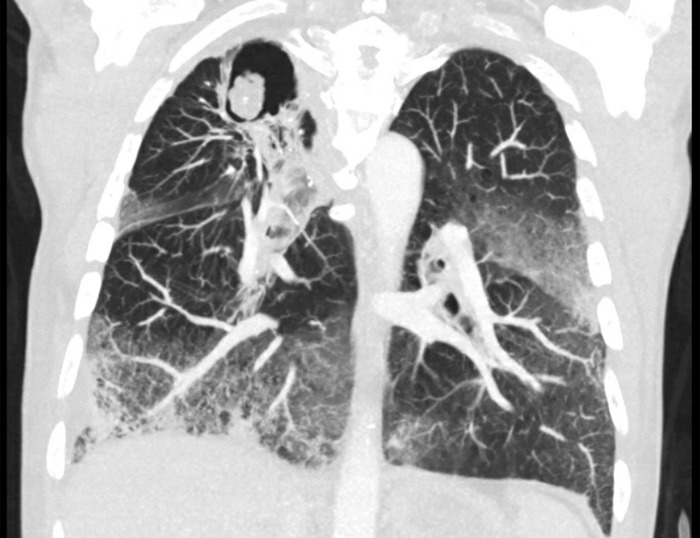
Admission chest computed tomography, coronal view, shows increased mediastinal lymphadenopathy, likely reactive.
